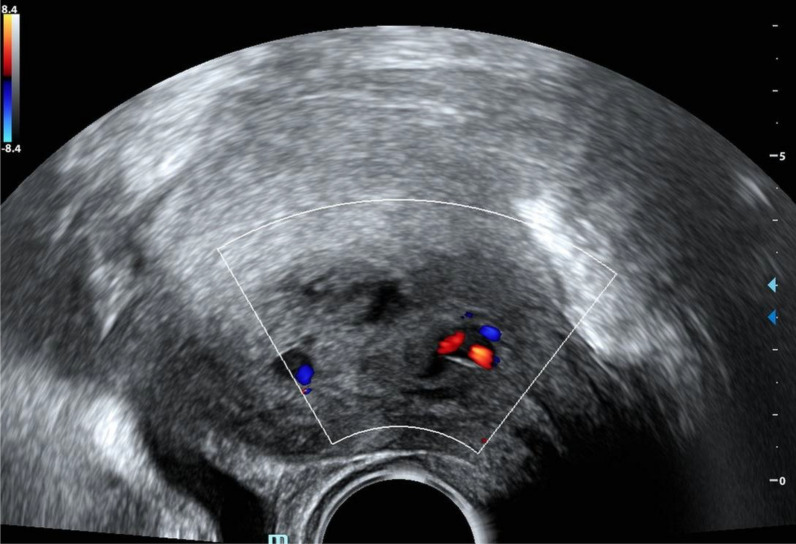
Transvaginal ultrasound at admission demonstrating an intrauterine clot with areas of perfusion
